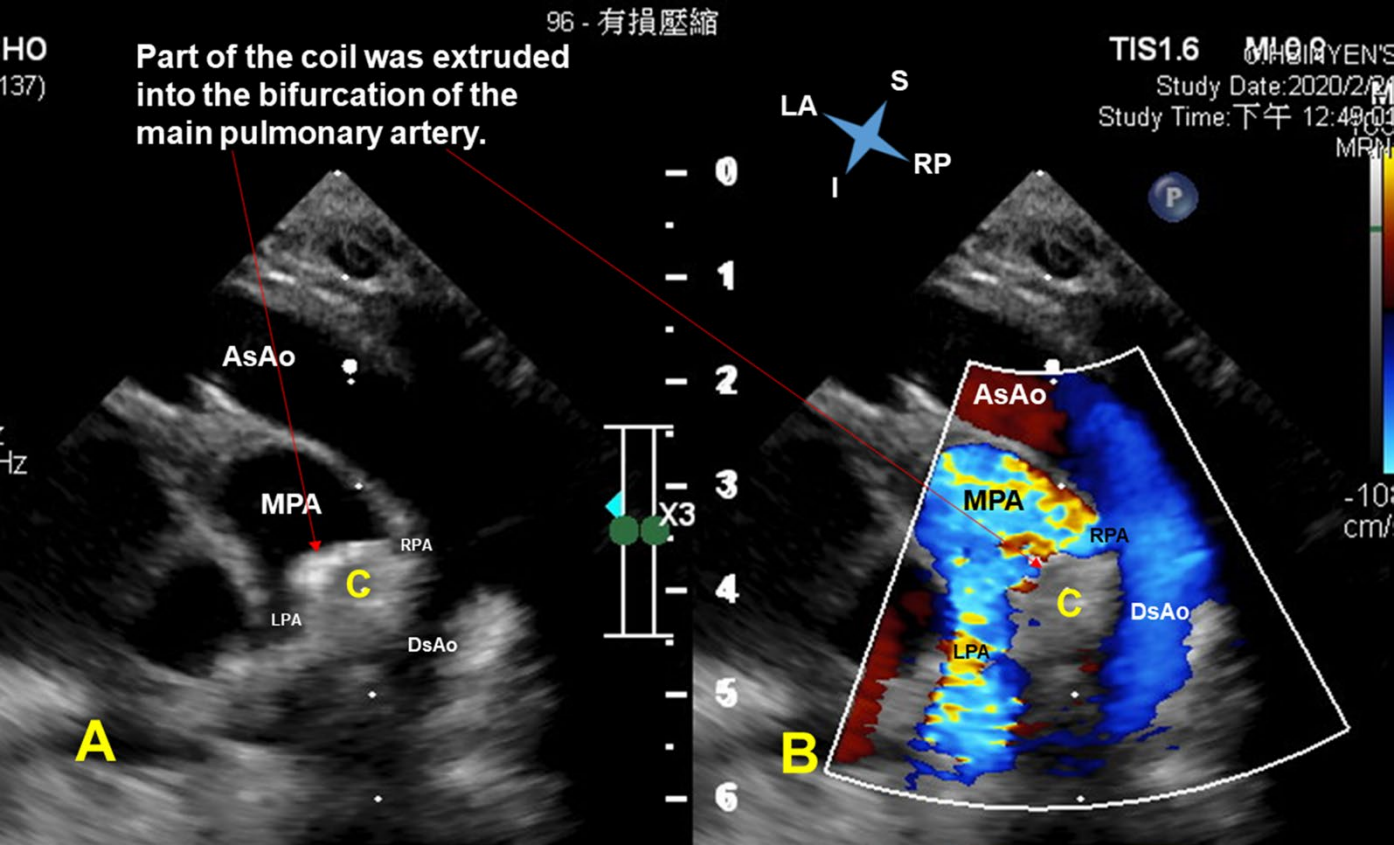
Suprasternal long-axis view with the transducer rotated and oriented to the right scapular tip (simultaneous 2-dimensional [a] and color flow Doppler [b] recordings) revealed right aortic arch without residual ductal shunting, but part of the coil was extruded into the bifurcation of the main pulmonary artery, in addition to the high-velocity mosaic pulmonary flow jet resulting from the previously performed pulmonary artery banding. It also showed the high-velocity pulmonary flow jet hit the extruded coil. *AsAo* ascending aorta; *C* coil; *DsAo* descending aorta; *I* inferior; *LA* left-anterior; *LPA* left pulmonary artery; *MPA* main pulmonary artery; *RP* right-posterior; *RPA* right pulmonary artery; *S* superior
